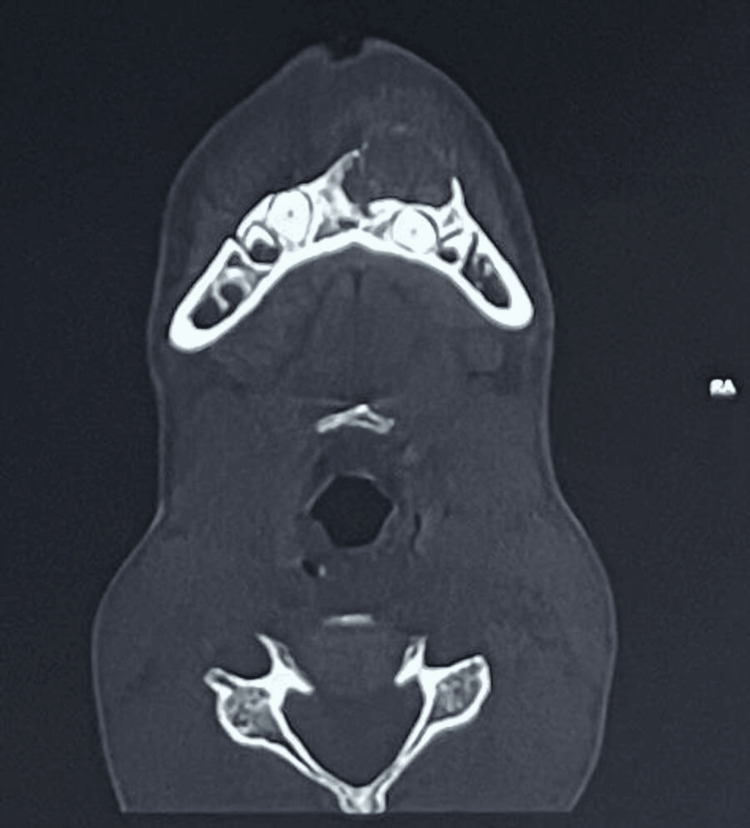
Axial view of CT scan showing an expansile intrabony lesion. Slice thickness: 1 mm at 1 mm interval.
